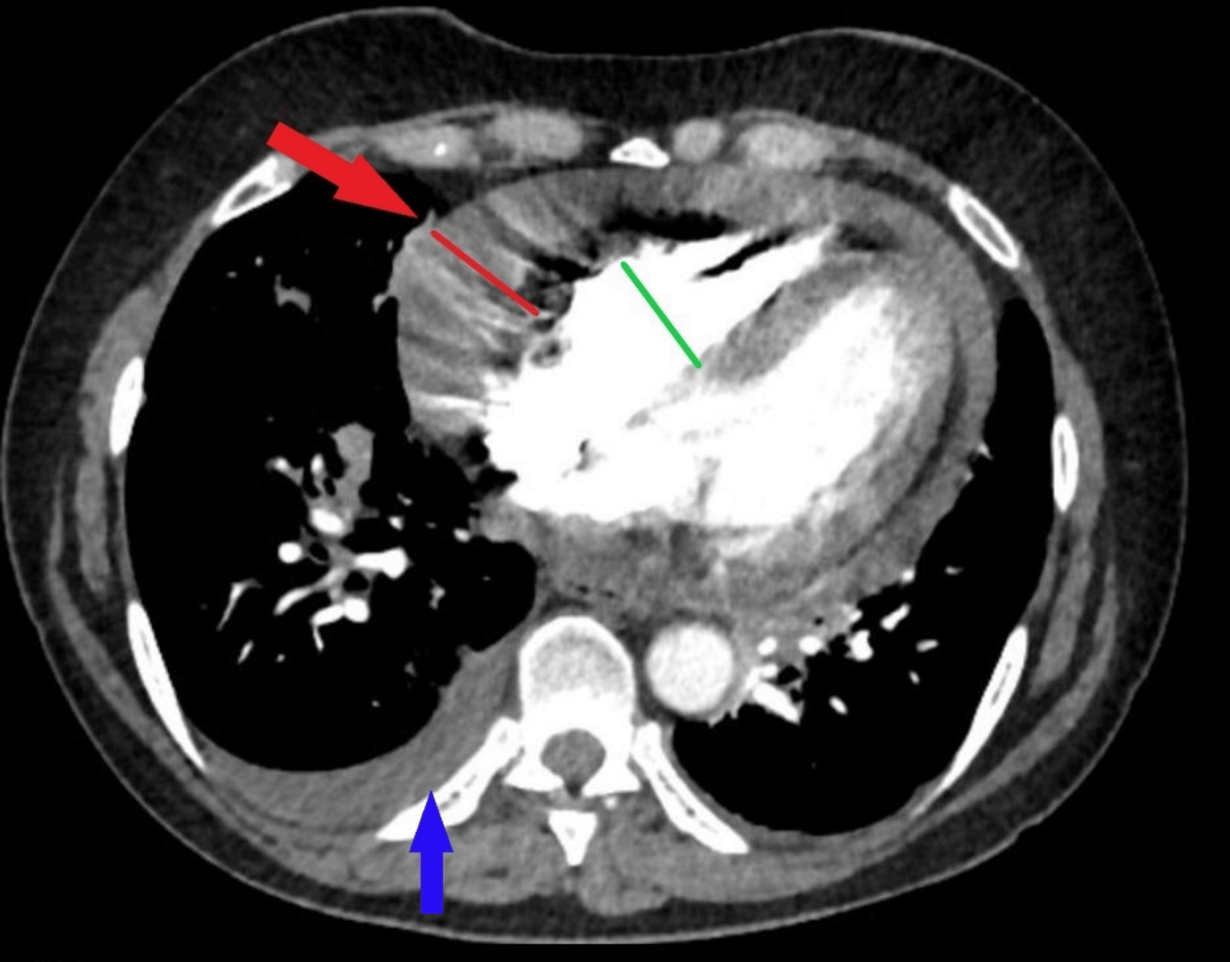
CT angiography study of the chest revealing a moderate to large pericardial effusion (red arrow and line), right-side pleural effusion (blue arrow), and collapsed right ventricle (green line) concerning for a tamponade.
